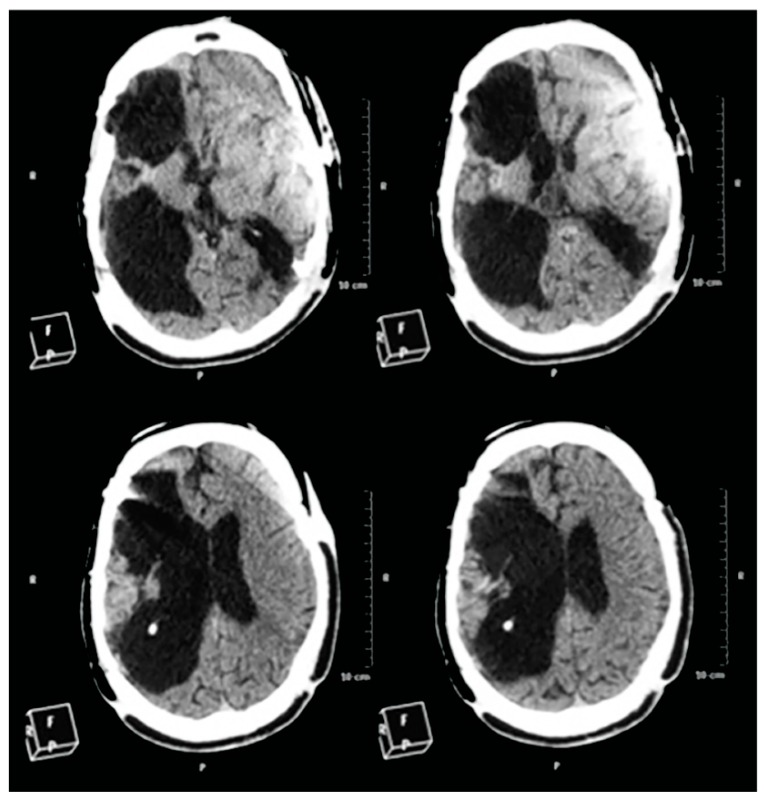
Two years of treatment. Despite the clinical improvements, a new CT-Scan revealed that the brain injury was higher than that observed in the first days after the accident and in the CT-Scan carried out two months later. Injuries in the right hemisphere were increased and new injuries appeared on the left one. Scale bar: 12 cm. F: Front. R: Right: P: Posterior.
